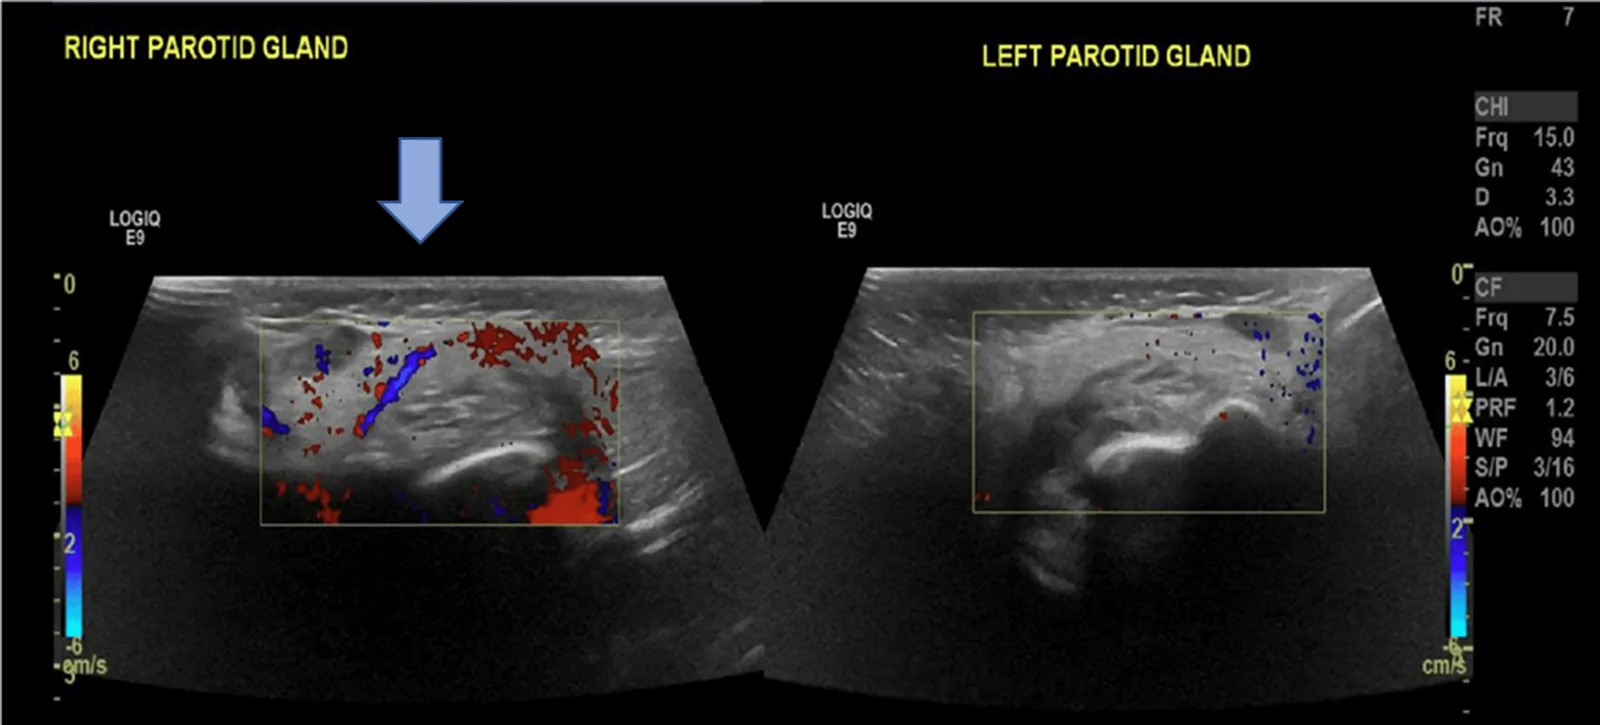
Ultrasound of the right parotid gland demonstrating increased vascularity and heterogeneous echotexture, consistent with recurrent parotitis
